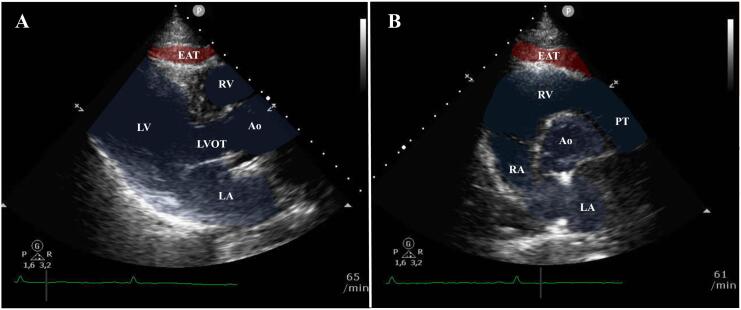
Epicardial Adipose tissue was quantified using transthoracic echocardiography. The region of interest was the end systolic space between the epicardial wall of the myocardium and the visceral layer of the pericardium in parasternal long (A) and short axis (B).
